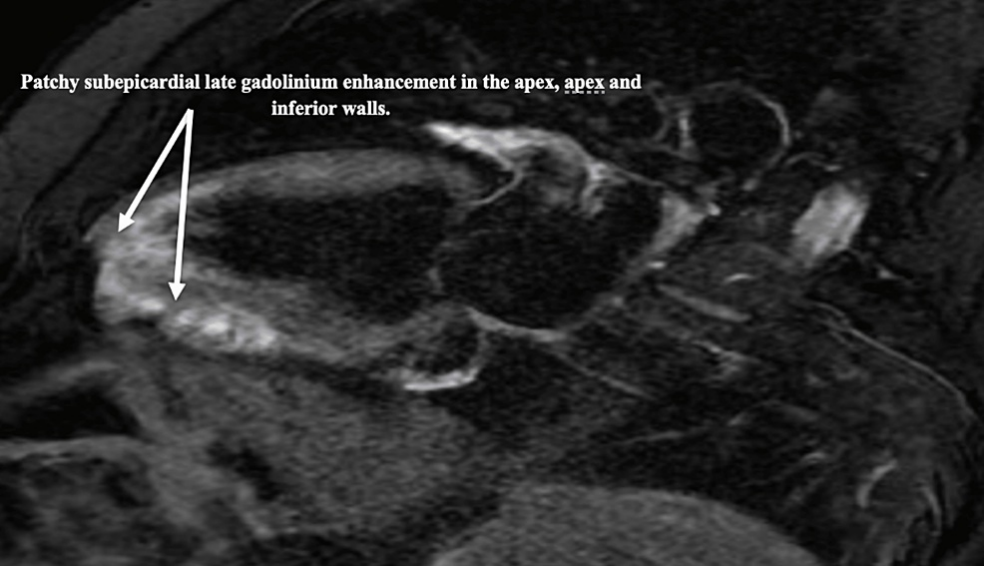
Cardiac MRI - two chambers view showing patchy subepicardial late gadolinium enhancement in the apex and inferior walls. MRI: magnetic resonance imaging
